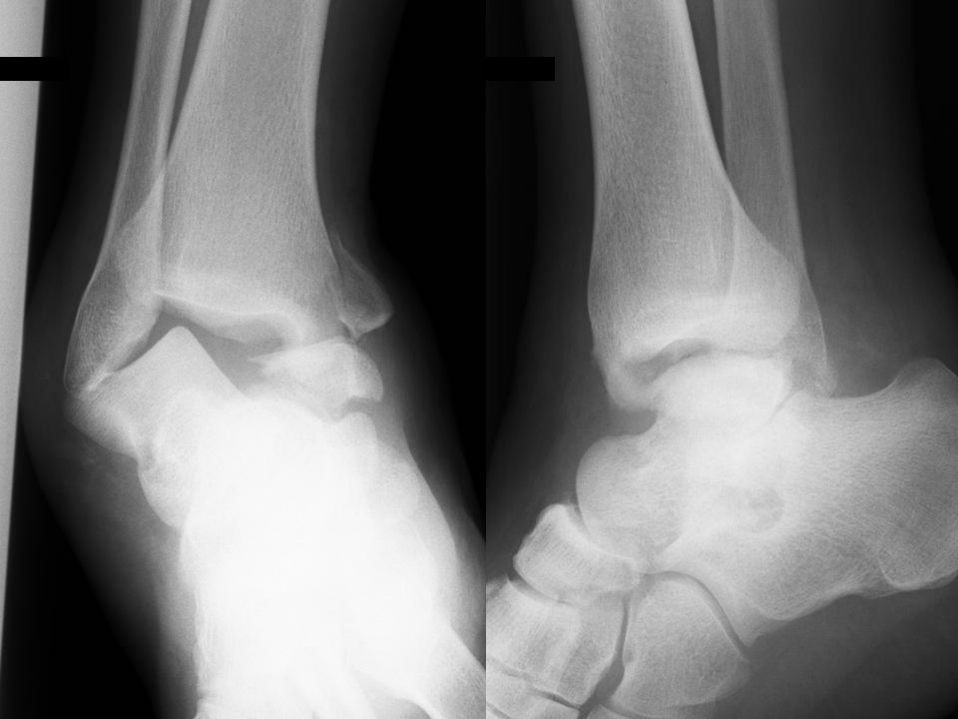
Anterio-posterior and lateral radiograph at time of presentation.
